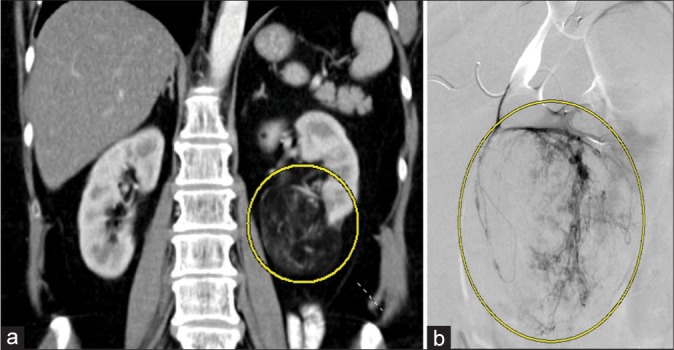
A 64-year-old woman with an incidental angiomyolipoma (AML). (a) Coronal computed tomography of the abdomen and pelvis demonstrating the AML (yellow circle) off the inferior pole of left kidney measuring 70 mm. (b) Digital subtraction angiography of a subsegmental renal artery demonstrating contrast enhancement of the AML off the inferior pole of the left kidney.
